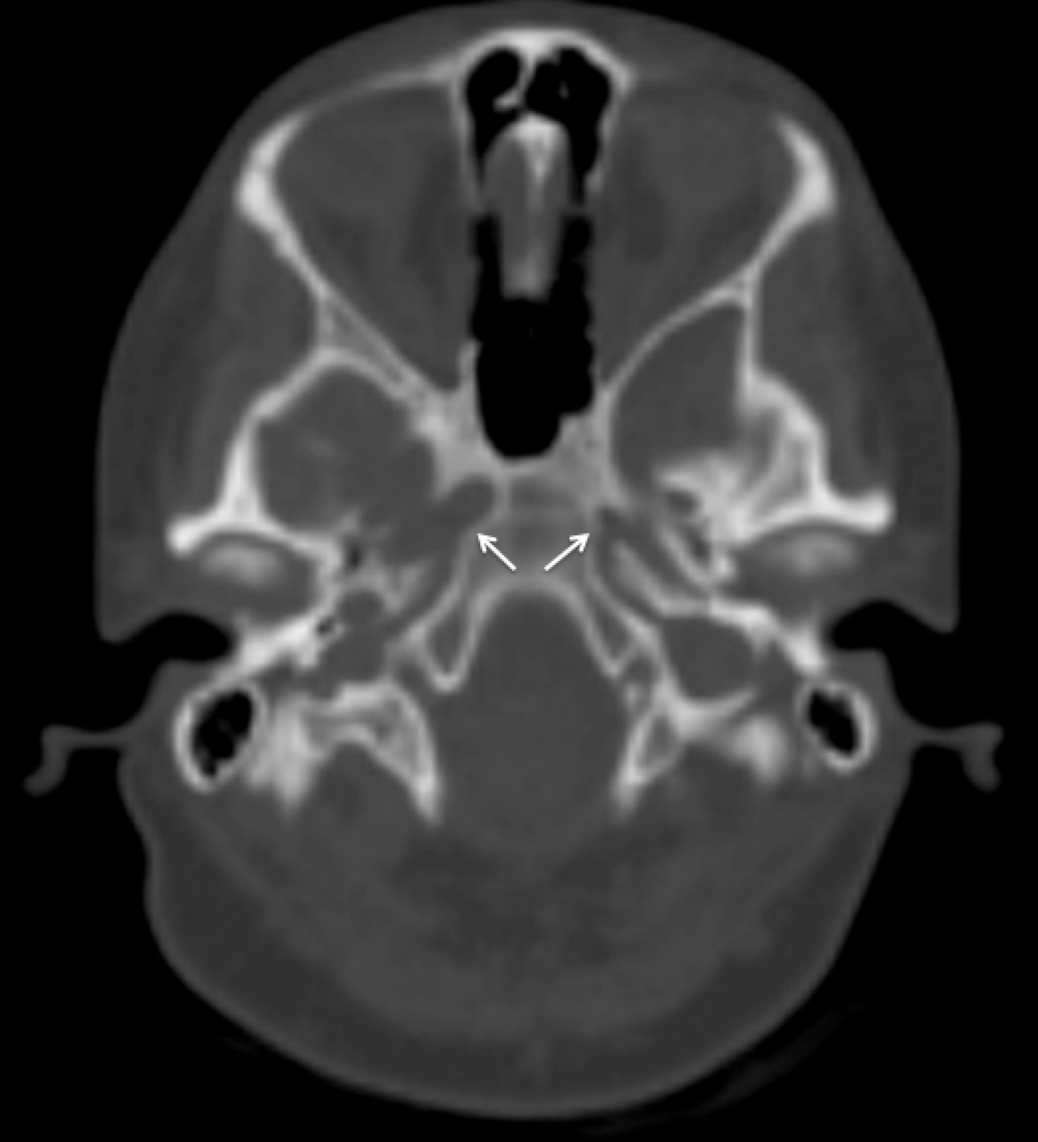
Axial CT The right carotid canal and left hypoplastic carotid canal are demarcated by arrows.
